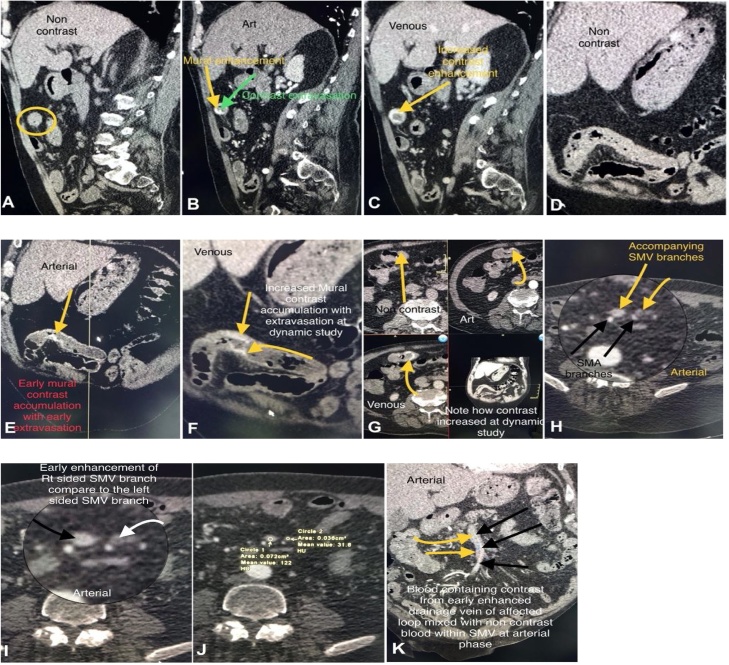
CT abdomen with contrast.
